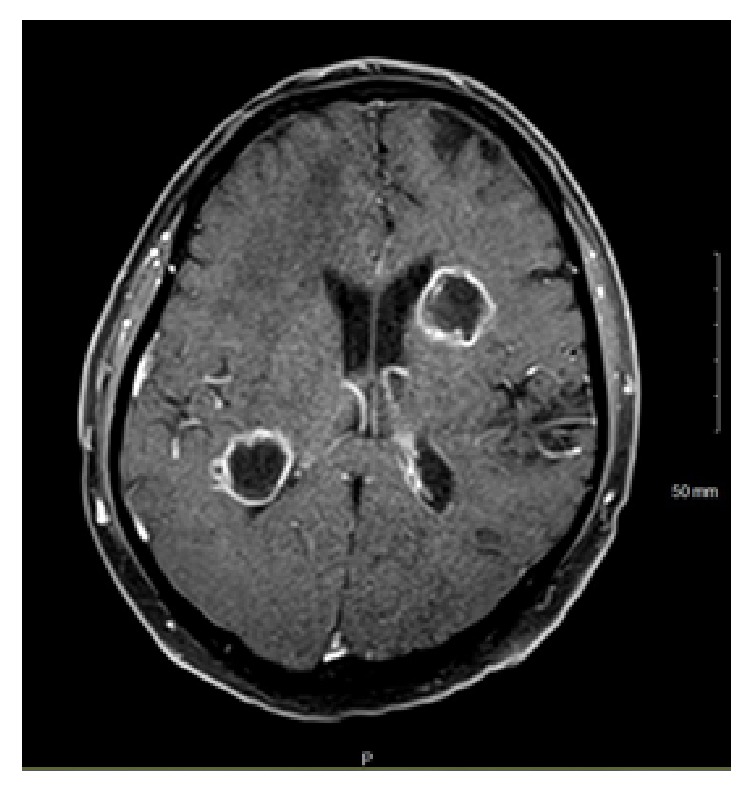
Axial contrast enhanced T1 WI brain MRI demonstrating rim enhancing lesions in the right peritrigonal white matter and left corona radiata.
